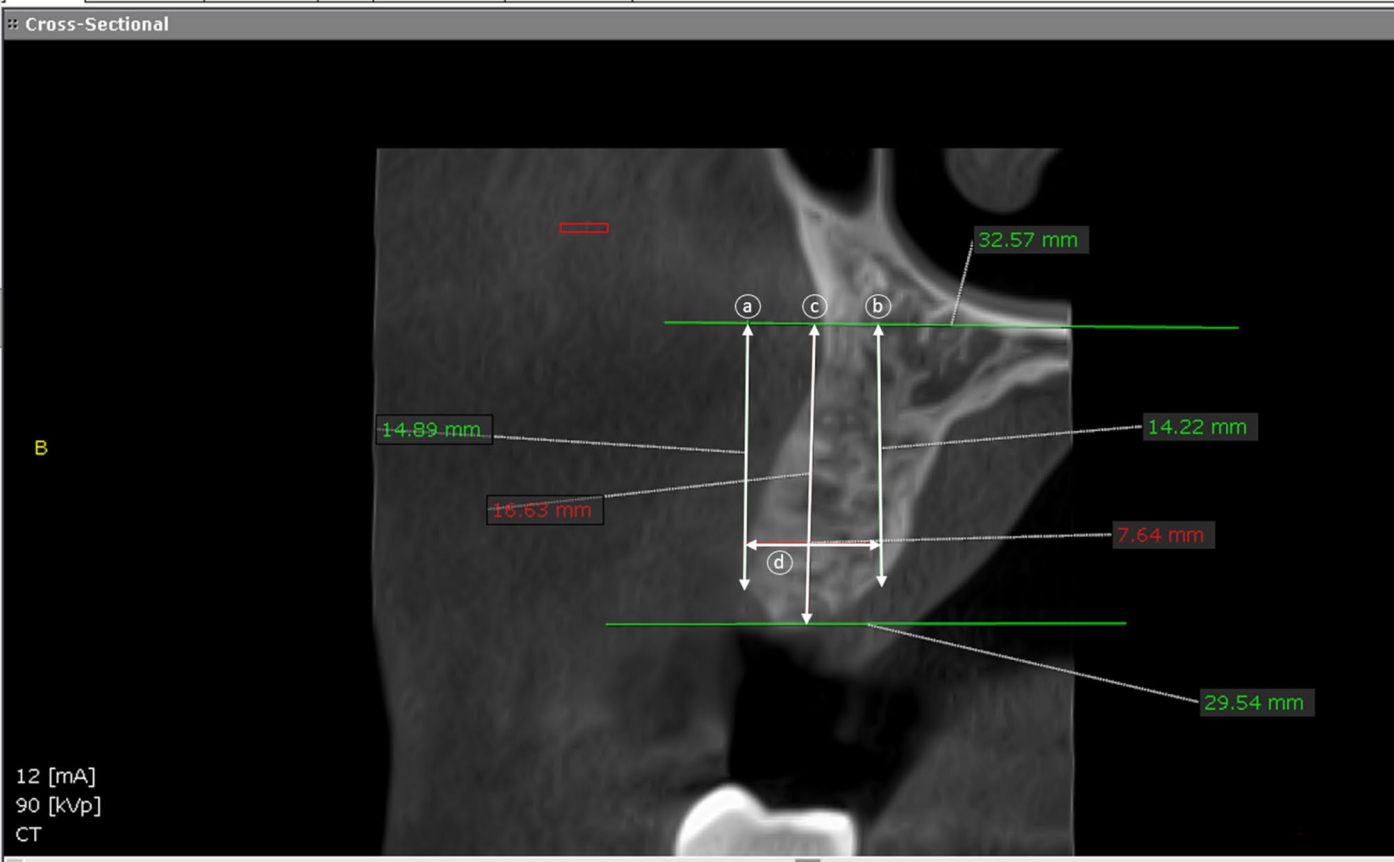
Radiographic measurement of bone width and height 6 months after surgery. (a) The measurement from the floor of the maxillary sinus to the top of the buccal alveolar bone (b) The measurement from the floor of the maxillary sinus to the top of the palatal alveolar bone (c) The measure from the floor of the maxillary sinus to the halfway between the palatal and buccal plates of bone. (d) The bone width is achieved by measuring the distance from points (a) to (b)
